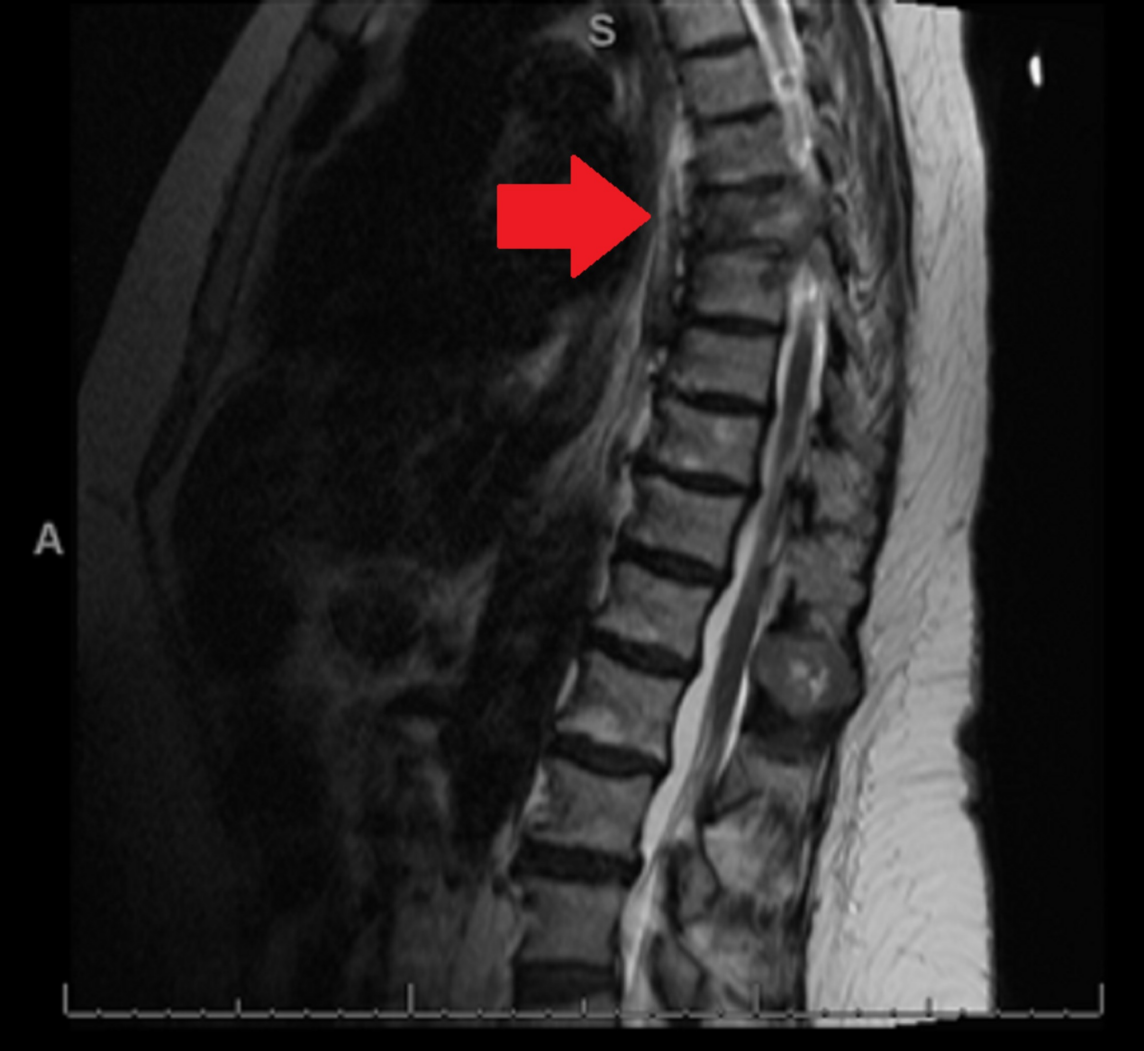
Thoracic spine lesions via magnetic resonance imaging T2 weighted magnetic resonance image demonstrating thoracic spine lesions. Red arrow demonstrating T7 vertebral body pathologic fracture and collapse.
